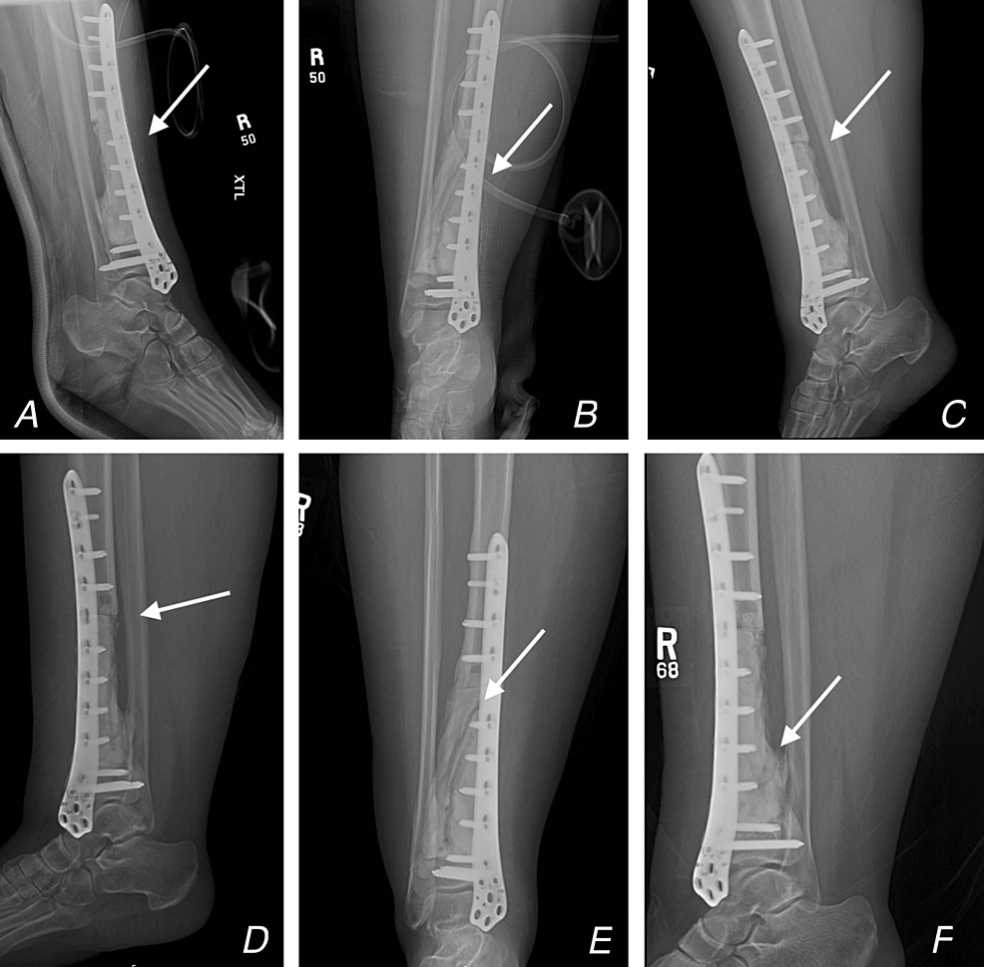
Post-operative X-ray images A: A lateral view of the right distal tibia and ankle X-ray on post-operation Day 1,  B: An antero-posterior (AP) view of the right distal tibia and ankle X-ray on post-operation Day 1,  C: Lateral view four months post-operation, D: Lateral view 10 months post-operation, E: AP view 14 months post-operation, F: Lateral view 14 months post-operation. Arrows showing fixation and the progressive bone cement integraion.
